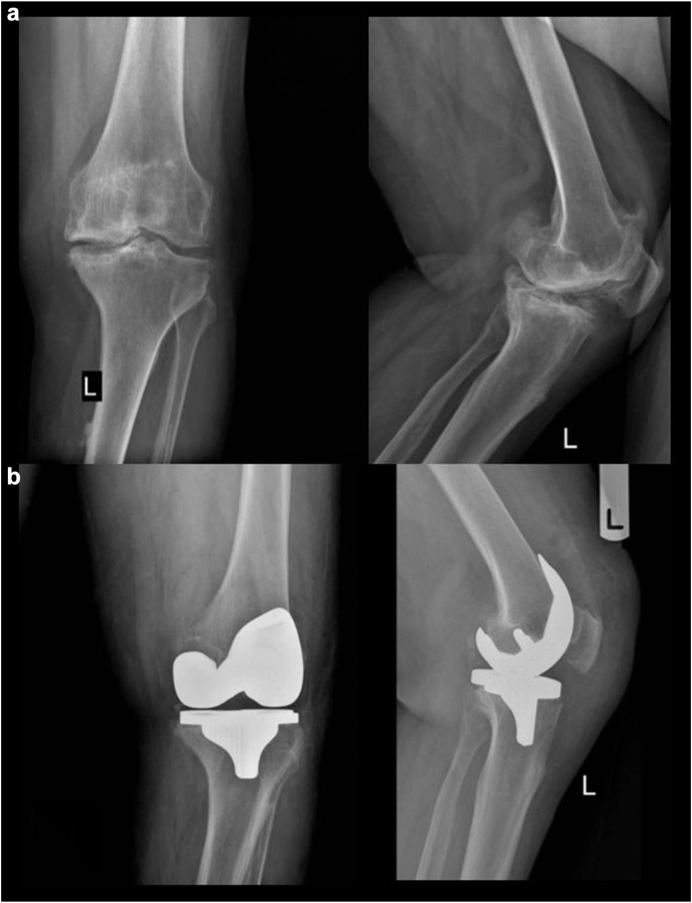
(a) Preoperative anteroposterior and lateral radiographs of the left knee in case 3 reveal advanced tricompartmental osteoarthritis characterized by pronounced joint space obliteration, marginal osteophyte formation, and subchondral sclerosis. (b) Anteroposterior and lateral radiographs obtained 24 months postoperatively demonstrate a well-positioned left total knee prosthesis with desired alignment and no signs of loosening or mechanical complications.
